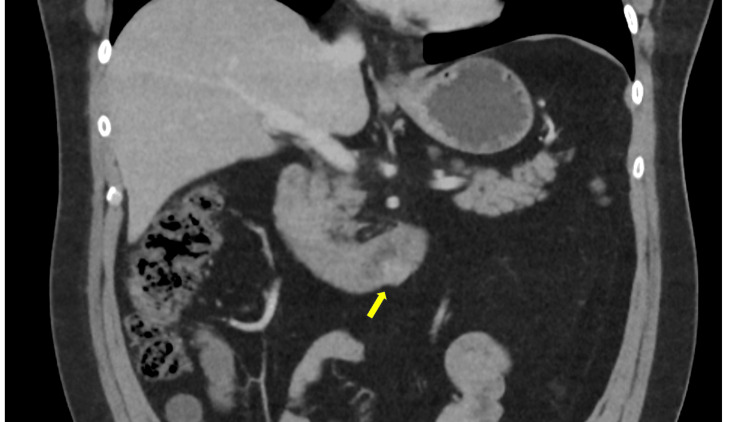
Case 1: Coronal CT view of ectopic pancreas. Contrast-enhanced CT of the abdomen, coronal view, demonstrating the well-defined subepithelial soft tissue lesion (yellow arrow) arising from the posterior wall at the junction of the third and fourth parts of the duodenum. The lesion shows homogeneous enhancement and projects into the lumen without associated obstruction or surrounding inflammatory changes. CT: computed tomography
